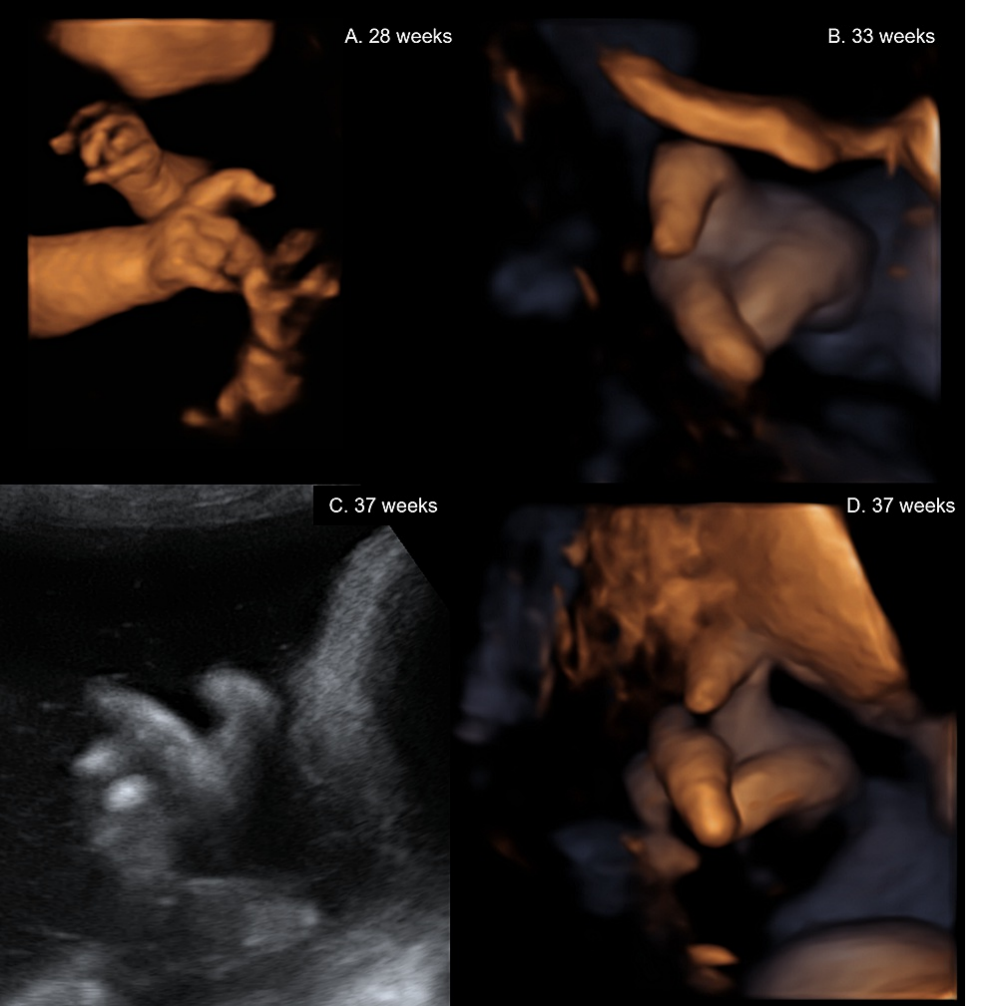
Clinodactyly on 2D and 3D ultrasound at different gestational ages (identified chronologically by letter)
